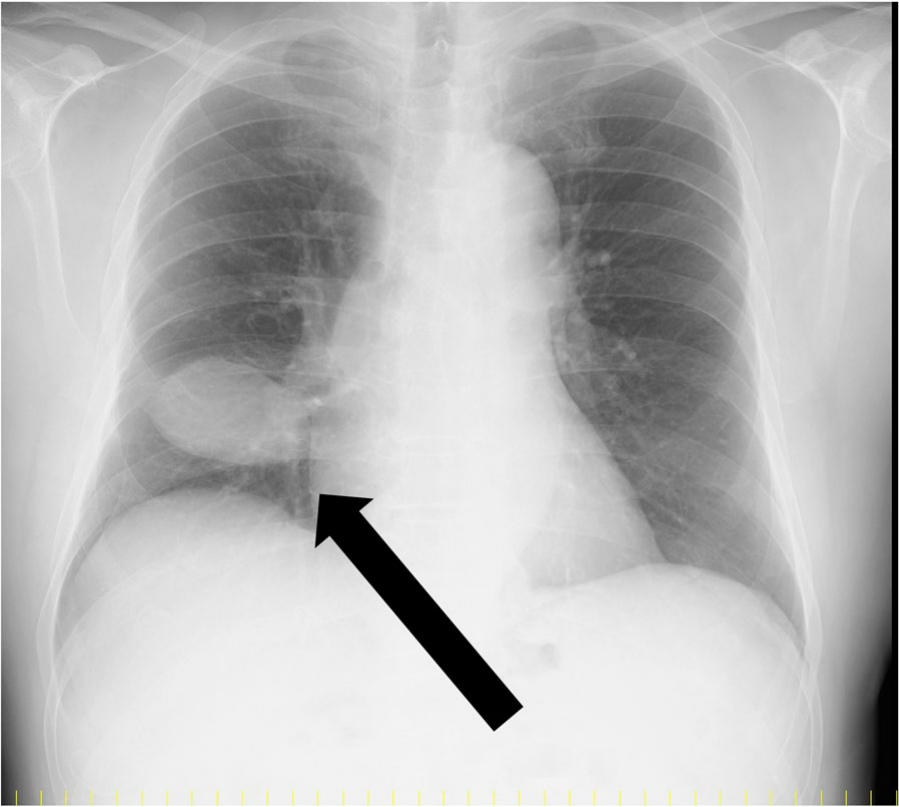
Chest radiograph (frontal view). Mass shadow in the middle and lower lobes of the right lung
